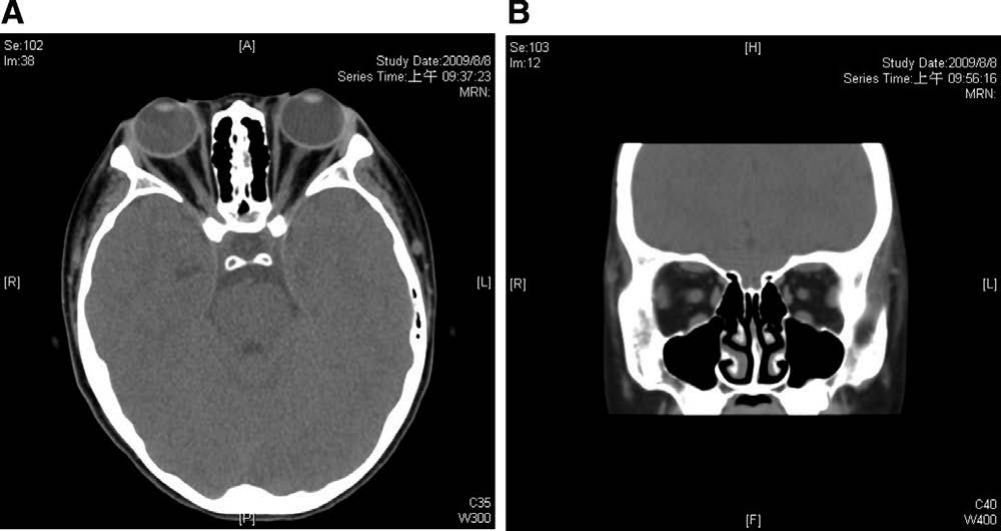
(A and B) The axial and coronal views of the orbital CT scan. CT = computed tomography.
